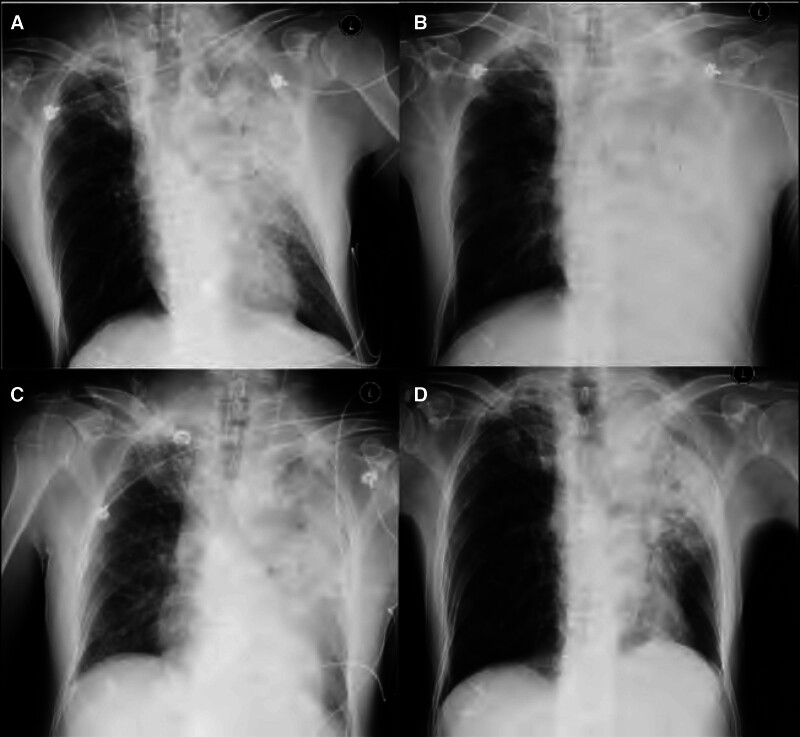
Simple radiographs during the course of VA-ECMO shown the progress of the lung infiltrates, consolidation and pleural effusion. (A) A simple radiograph on arrival at ICU shown severe infiltrate and consolidation in the left upper lung field and mild infiltrate in the right apex pulmonis and left lower lung field. (B) A simple radiograph on day 2 shown severe infiltrate and consolidation in the left lung field and moderate infiltrate in the right apex pulmonis field and left pleural effusion. (C) A simple radiograph on day 5 shown severe infiltrate and consolidation in the left lung field, less severe infiltrate and consolidation in the left lower lung field and reduced left pleural effusion. (D) A simple radiograph on day 30 shown partial consolidation and fibration was on left upper lung. ICU = intensive care unit, VA-ECMO = veno-arterial extracorporeal membrane oxygenation.
